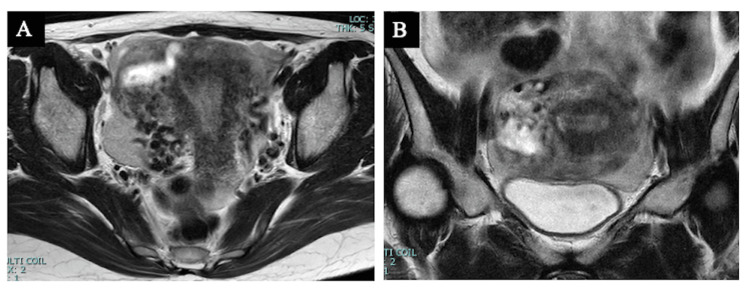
Pelvic contrast-enhanced magnetic resonance imaging (T-enhanced image) (A) Transverse plane; (B) Coronal plane; a mixed high-to-low signal intensity (5 cm) mass was found on the right side of the uterine body.
